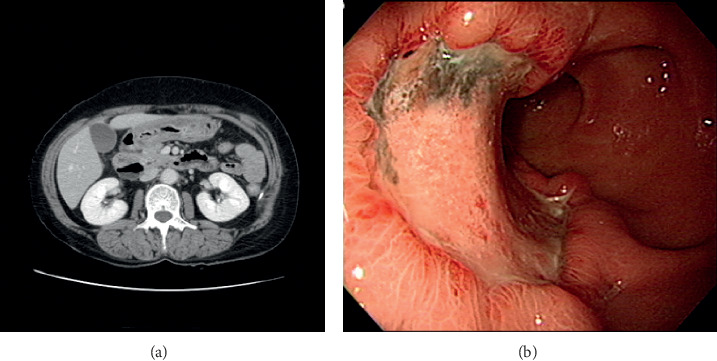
(a) Significant decreased size of hematoma in the stomach antrum and lower body compared with previous computed tomography. No visible active bleeding in stomach is observed. (b) Gastroscopy showing active ulcer on the anterior wall of the antrum and low body.
